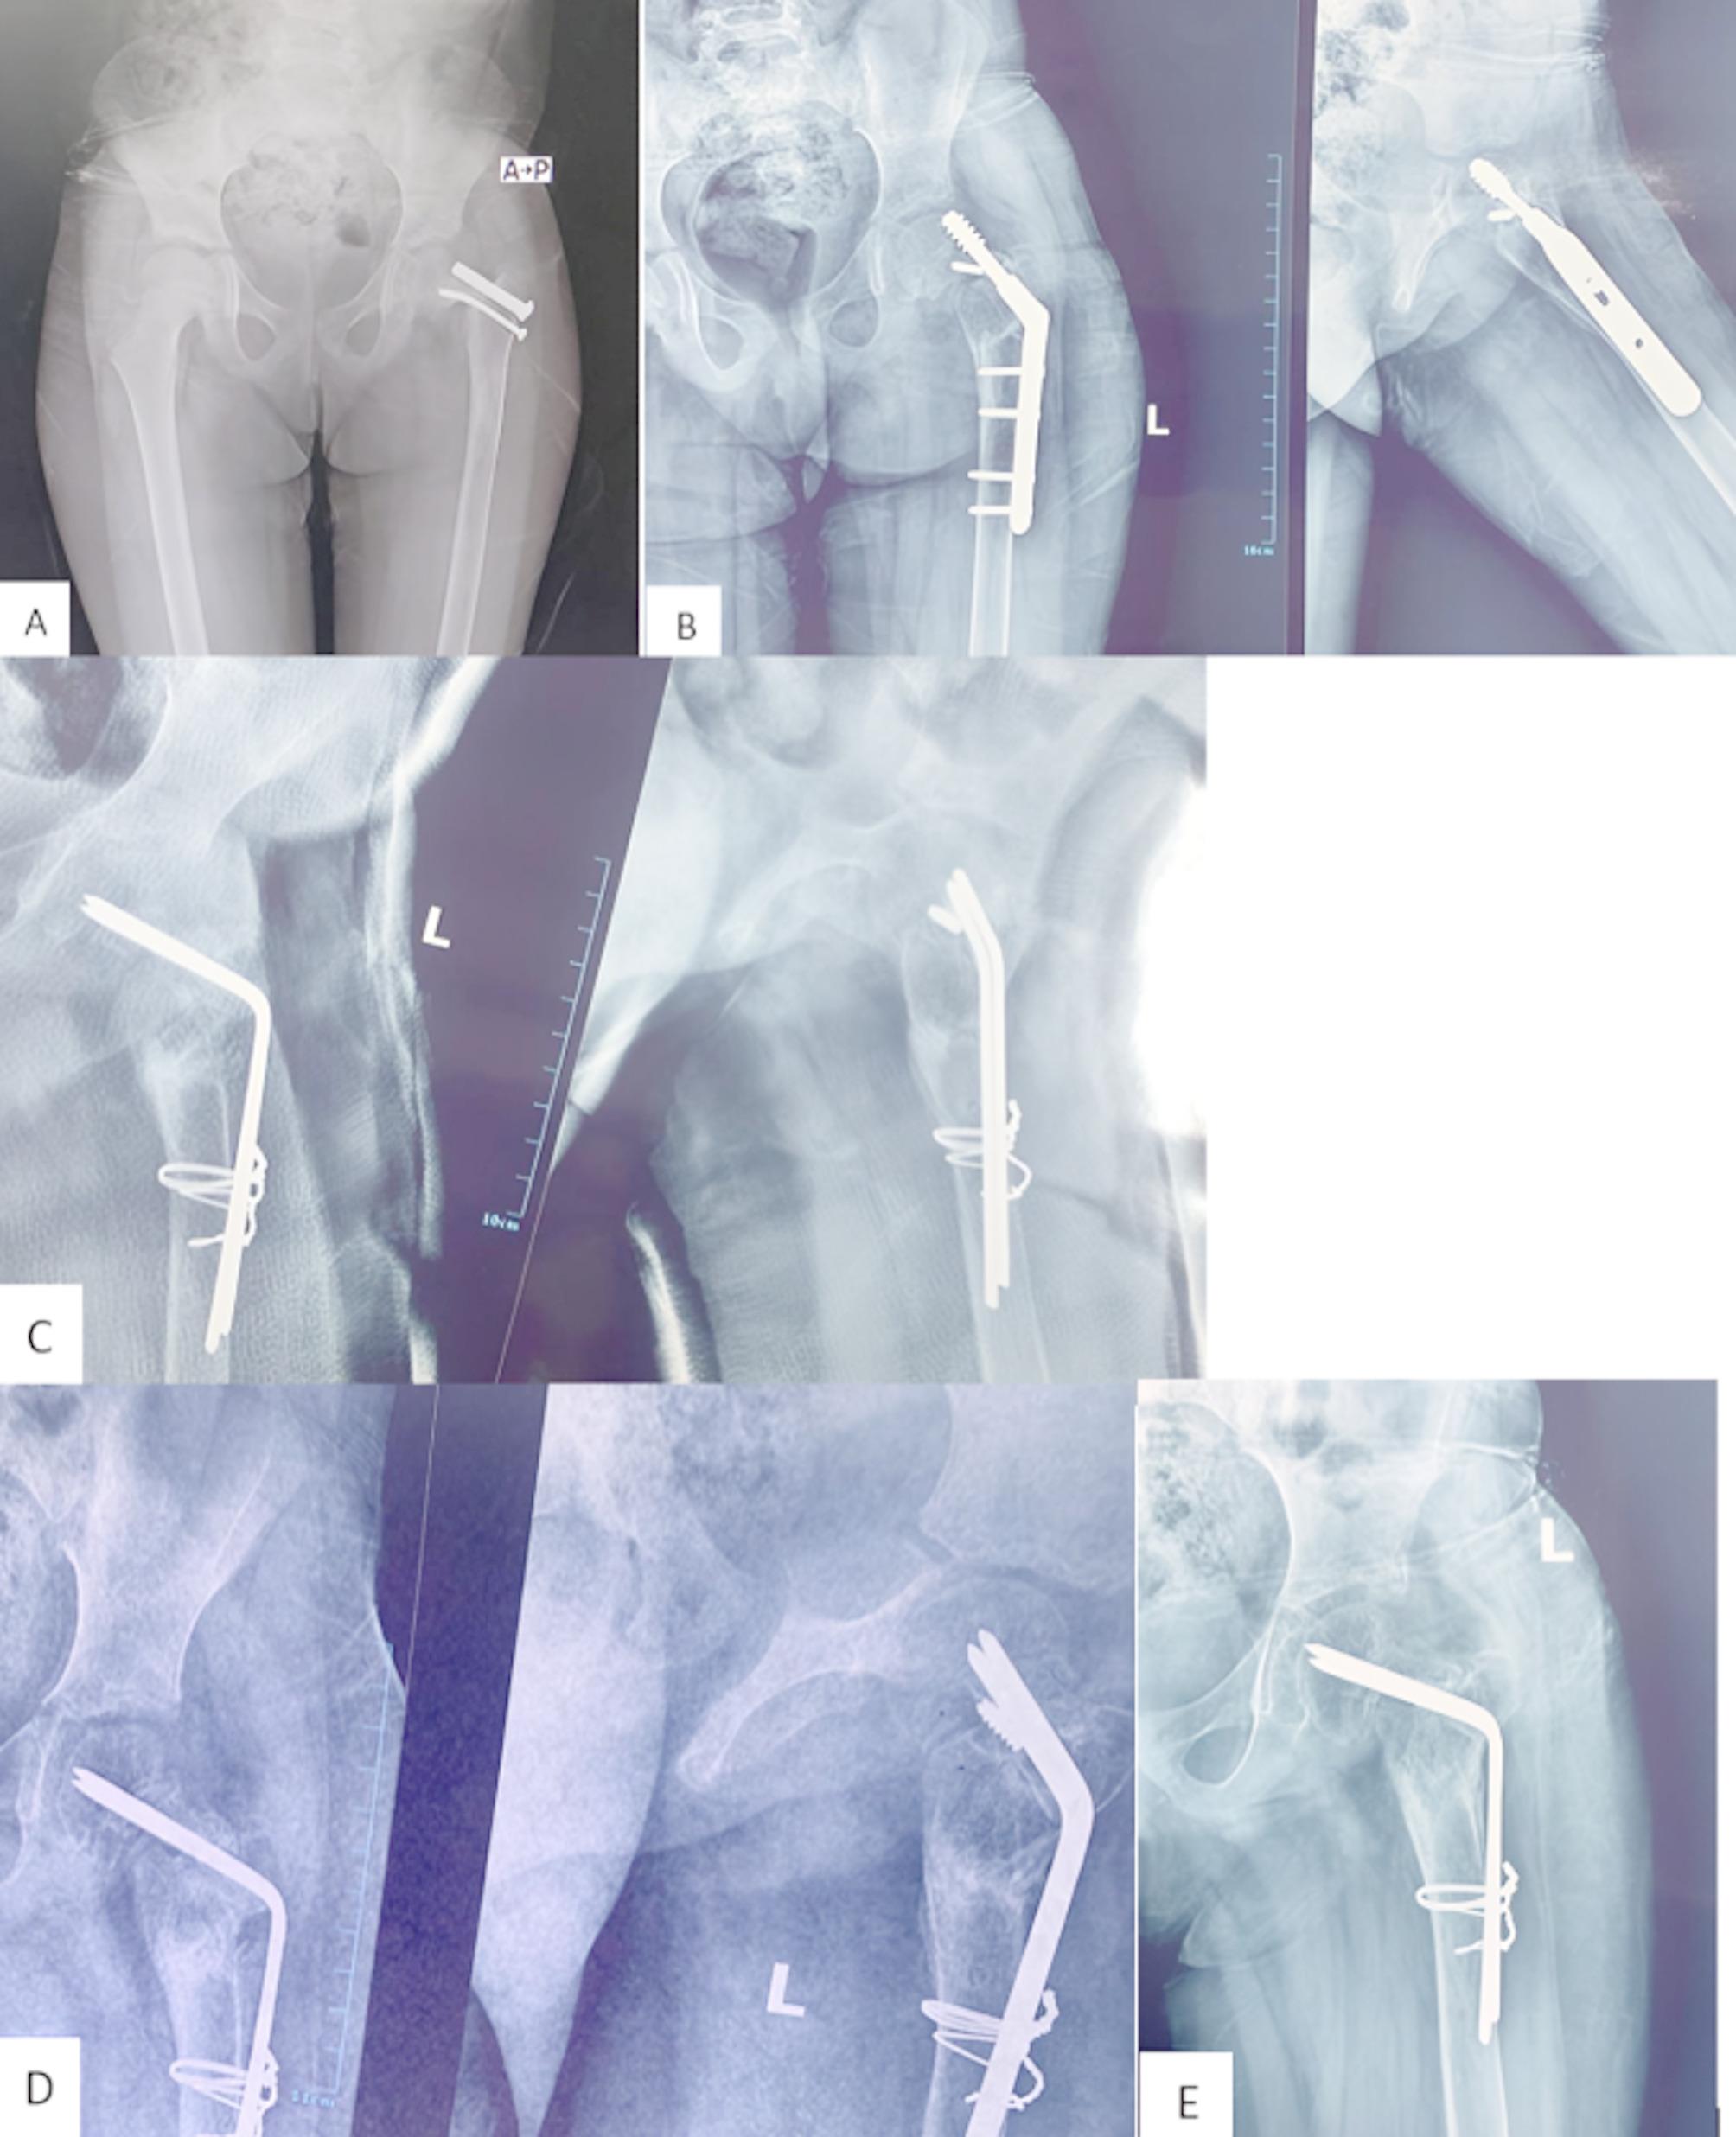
A 5-year-old girl with nonunited left femoral neck fracture and failed cannulated screws fixation. B: Six months later, she was then revised by a dynamic hip screw, that was complicated by a lag cut through and marked neck resorption. C, D: Finally, she was revised by removing the DHS and fixed by the Wagner technique with a fibular strut graft; C: early post-operative 4 months after surgery and D: Eight months after surgery. E: X-ray of the left hip shows united femoral neck fracture and united subtrochanteric osteotomy
